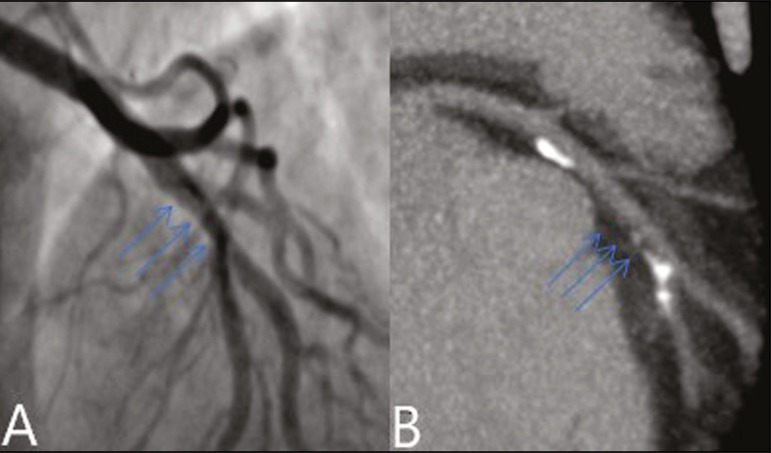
Mixed plaque, resulting in discrete stenosis in the ADA (CAD-RADS 2). A: Analysis by catheterization. B: Analysis by CTA.
